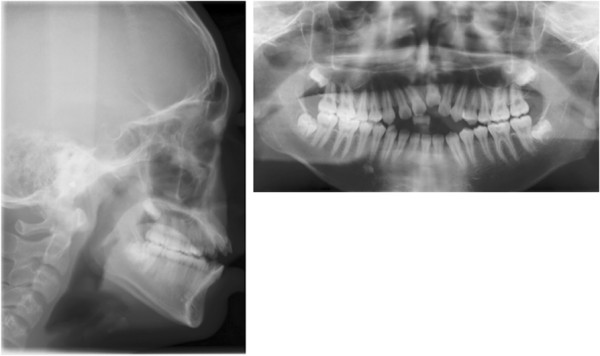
Panoramic and cephalometric radiographs before treatment.
